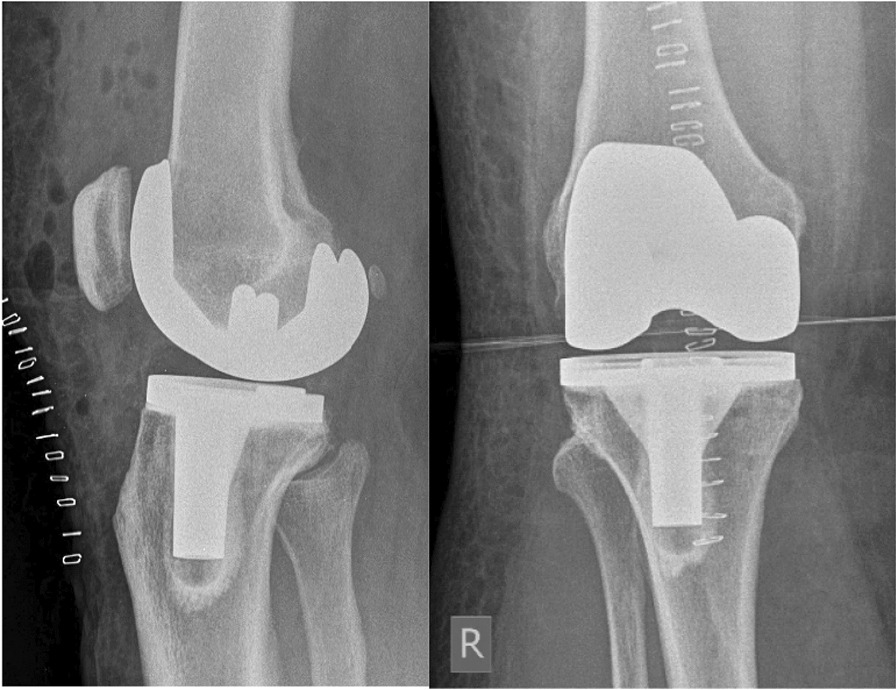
Postoperative X-ray control following the implantation of right total knee arthroplasty
